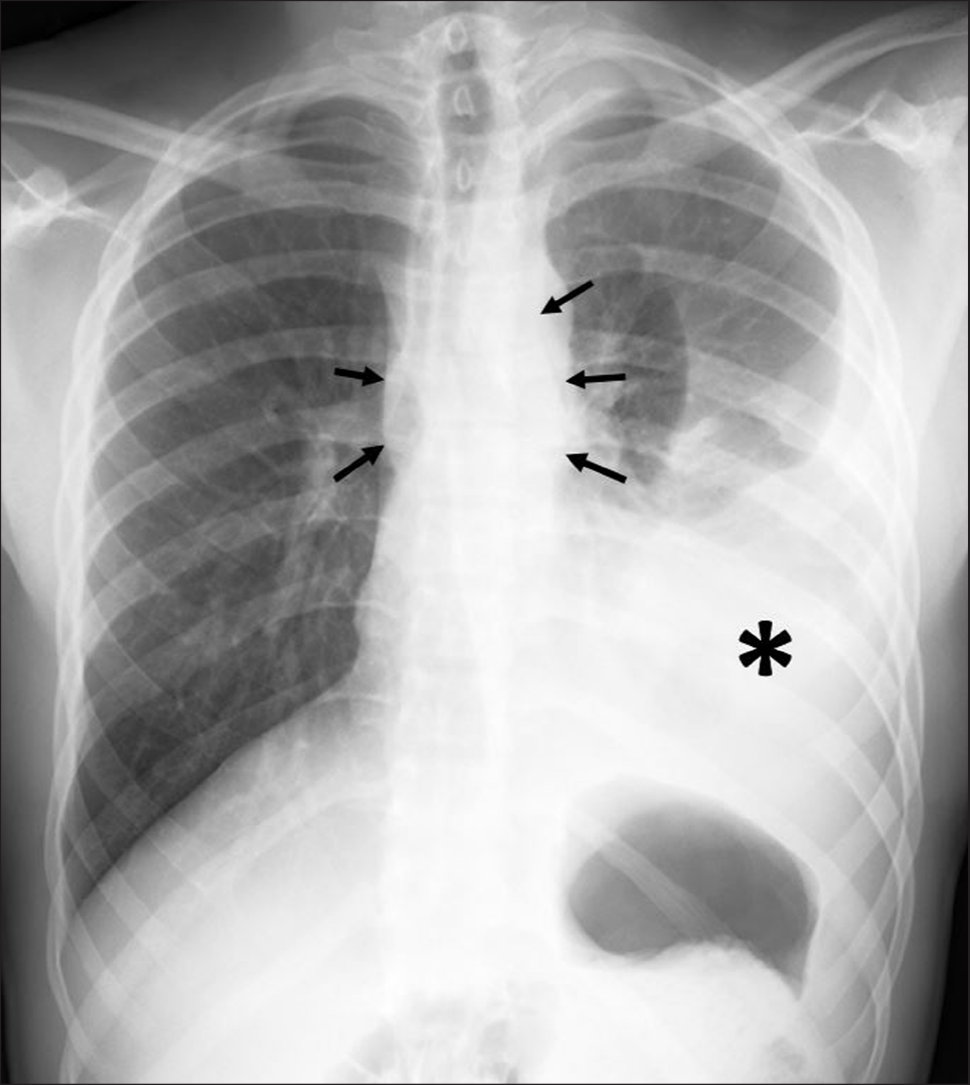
Postero-anterior (PA) chest radiograph showing large left pleural effusion (asterisk) and bilateral bulging of paraspinal lines (arrows).
